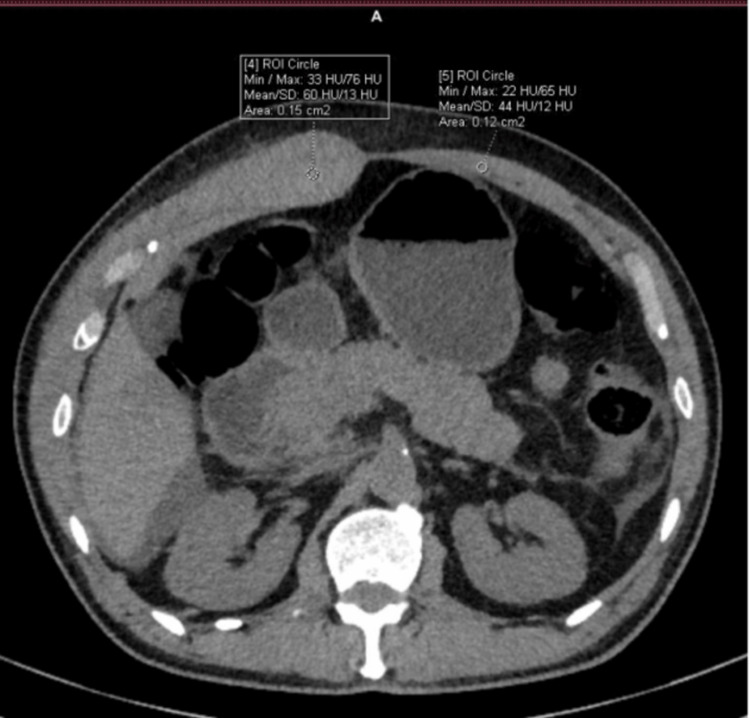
Non contrast CT image of subject showing abdominal wall CT: computed tomography
